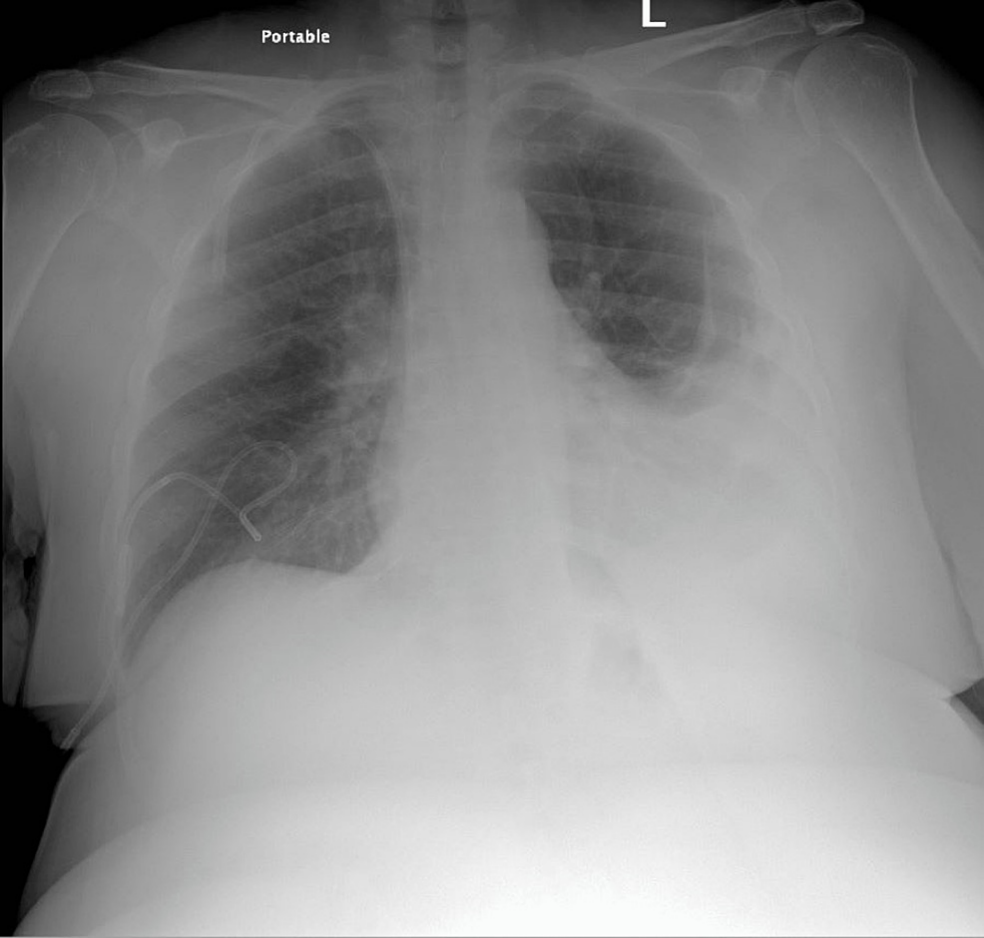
Chest x-ray of our patient demonstrating a left sided pleural effusion
